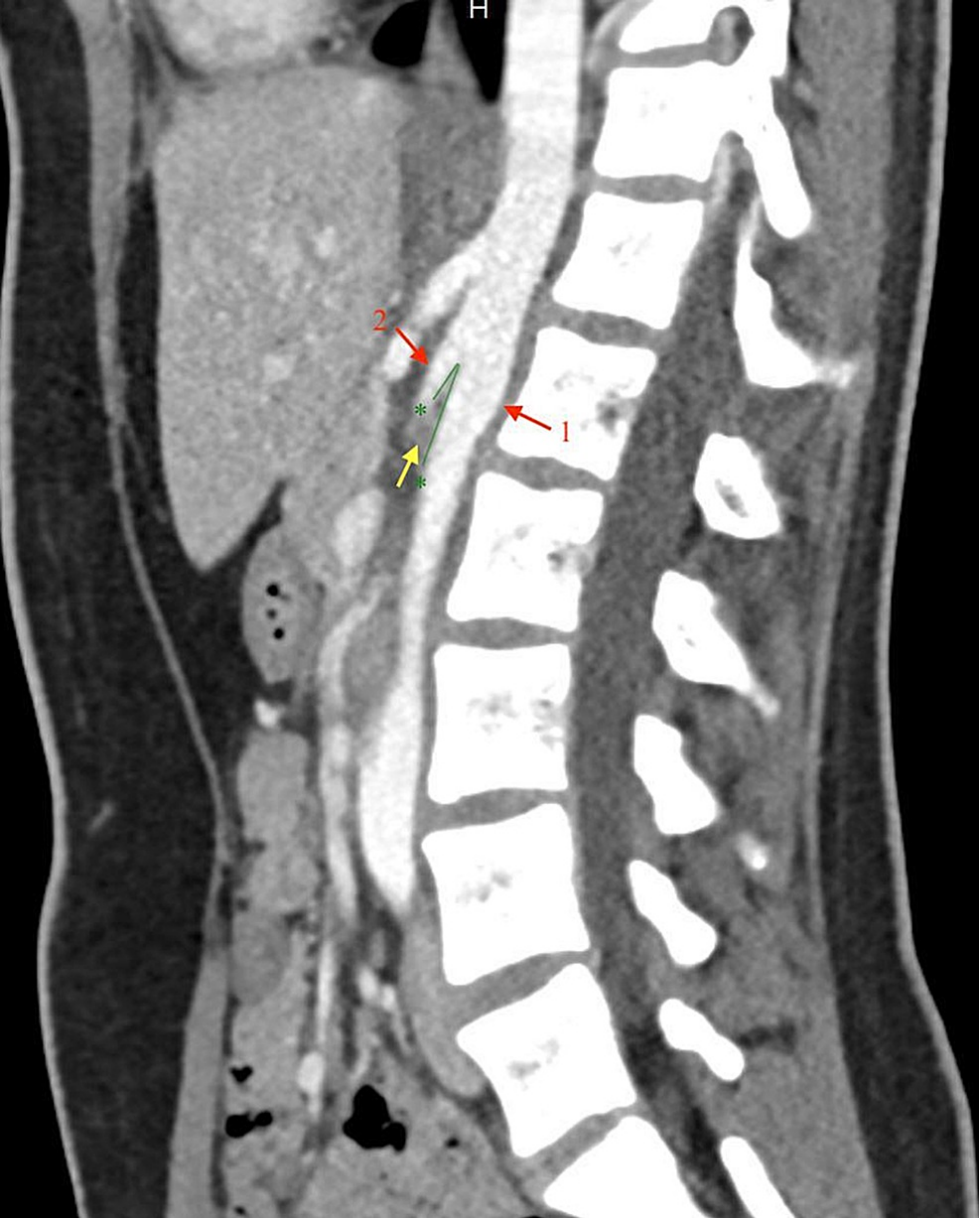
Sagittal CECT shows the aortomesenteric angle (green lines) of 11 degrees. Note resultant compression of the LRV (yellow arrow) between the aorta (1) and the SMA (2). CECT - Contrast-enhanced computed tomography; LRV - left renal vein; SMA - superior mesenteric artery
